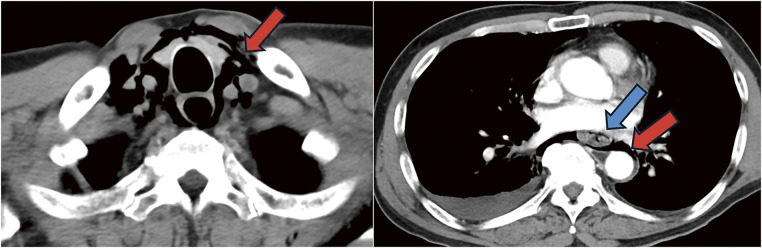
Computed tomography (CT) scan. CT revealed pneumomediastinum from the pharynx to the esophagogastric junction (the red arrow). The blue arrow shows the thickness and the disruption of the esophageal wall.
